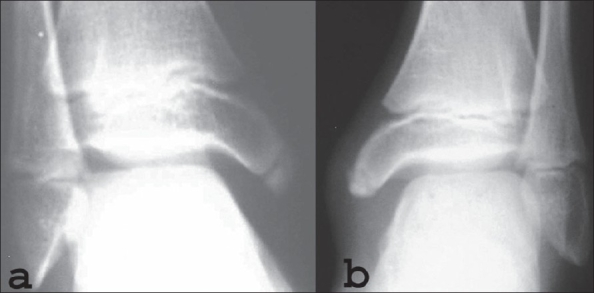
Anteroposterior X-ray of right (a) asymptomatic medial malleolus shows an accessory center. (b) Anteroposterior X-ray of left symptomatic (b) side shows soft tissue shadow and fragmentation of the accessory center
